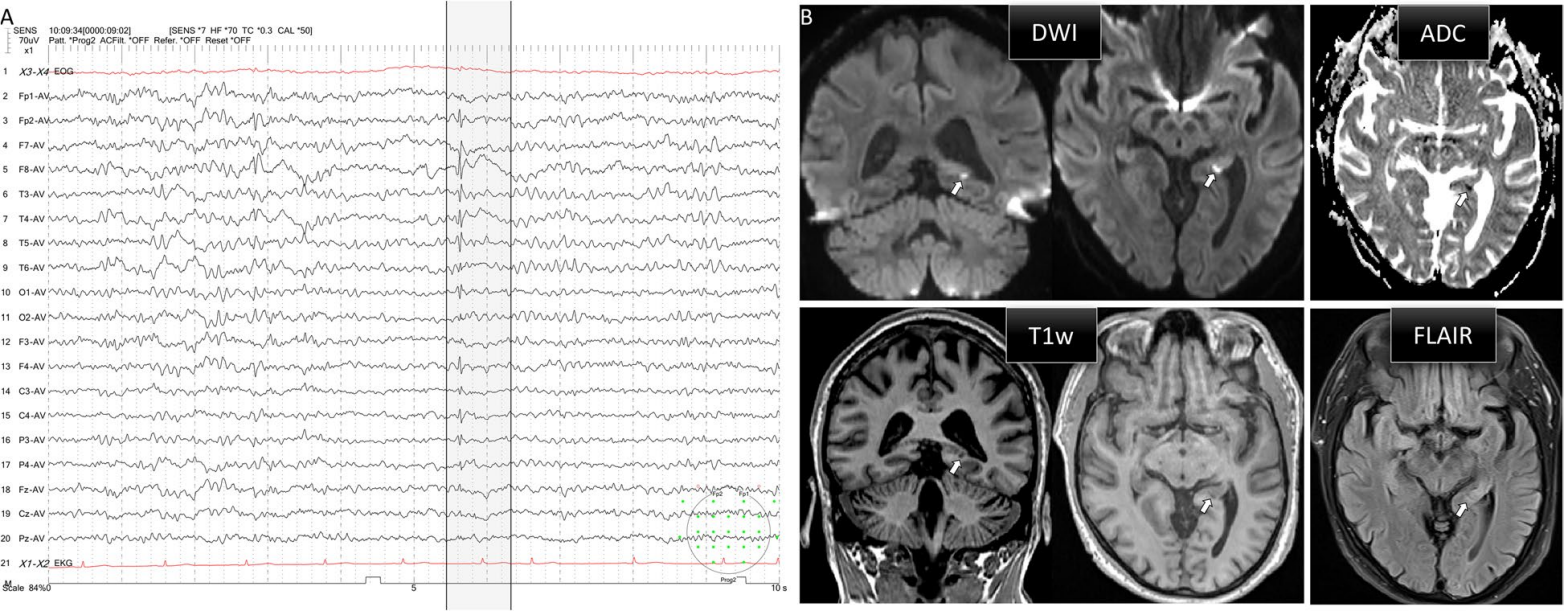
A Scalp electroencephalography recording on the day of admission 20 h after most recent amnesic episode. Highlighted is a right frontotemporal spike followed by a slow wave. Four seconds prior to this prominent transient, there is a beta wave followed by a low-amplitude spike in the left temporal region B Magnetic resonance imaging 48 h after first amnesic episode. White arrows indicate a punctate focus of restricted diffusion and low ADC signal (top row) in the tail of the left hippocampus. Signal intensity in the lesion is low on T_1_-weighted and high on T_2_-weighted (FLAIR) sequences (bottom row)
